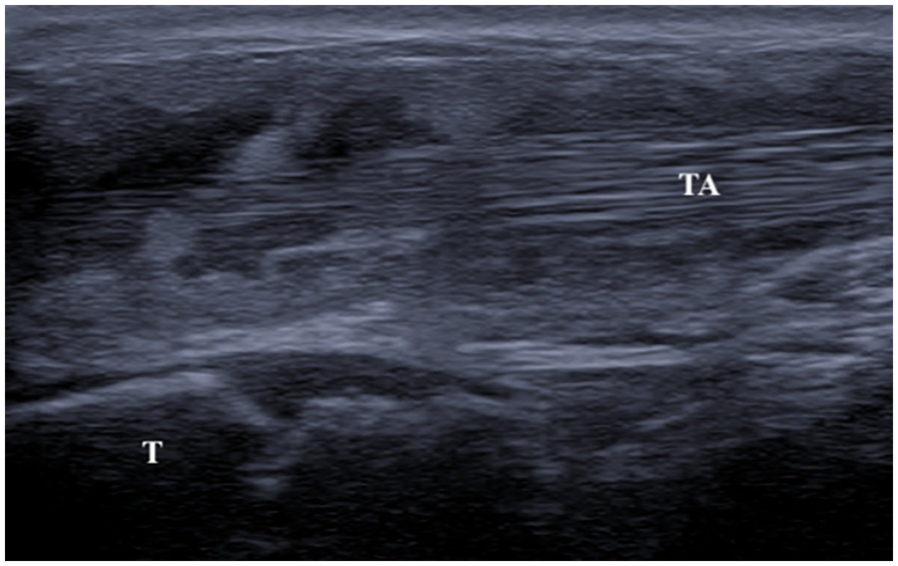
Ultrasound image (longitudinal scan) of anterior tibial tendon. Presence of anechoic peritendinous effusion and severe synovial hypertrophy with a villous pattern. (TA, tibialis anterior; T, tibia).
